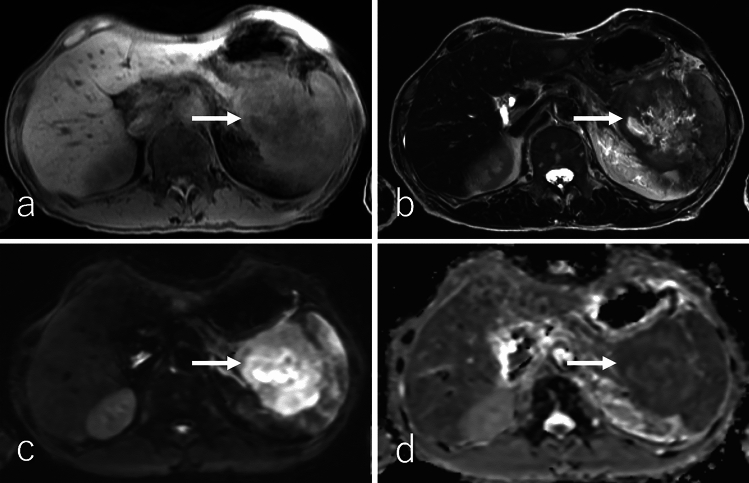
A 67-year-old male patient with adenosquamous carcinoma. a Fat-suppressed T1-weighted imaging (FS-T1WI), b T2-weighted imaging (T2WI), c diffusion-weighted imaging (DWI) with b value of 800 s/mm^2^, d apparent diffusion coefficient (ADC) map. The tumor is located in the pancreatic tail (arrow), measuring 8.0 cm in diameter, and demonstrates an expansile growth with well-defined margins. The tumor shows heterogeneous hypointensity on FS-T1WI and heterogeneous iso-to-hyperintensity on T2WI and DWI, and lower ADC values (1.09 × 10^−3^ mm^2^/s) relative to the pancreatic parenchyma
